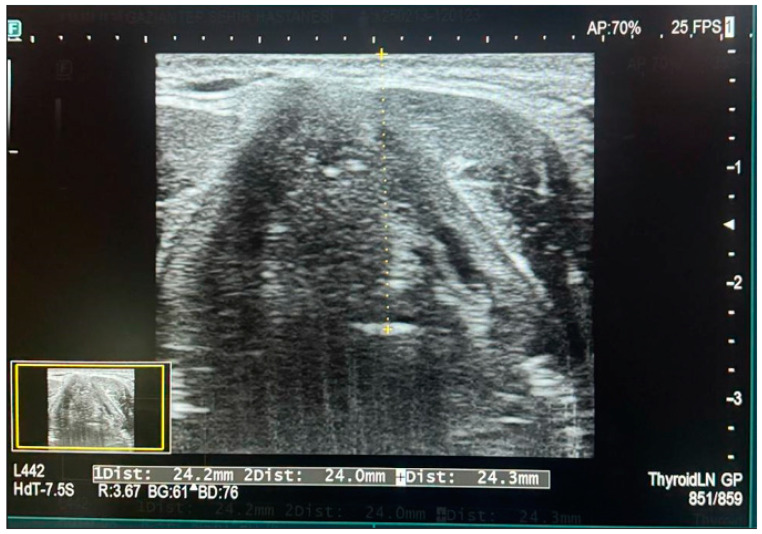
Measurement of skin-to-epiglottis distance via ultrasound.
